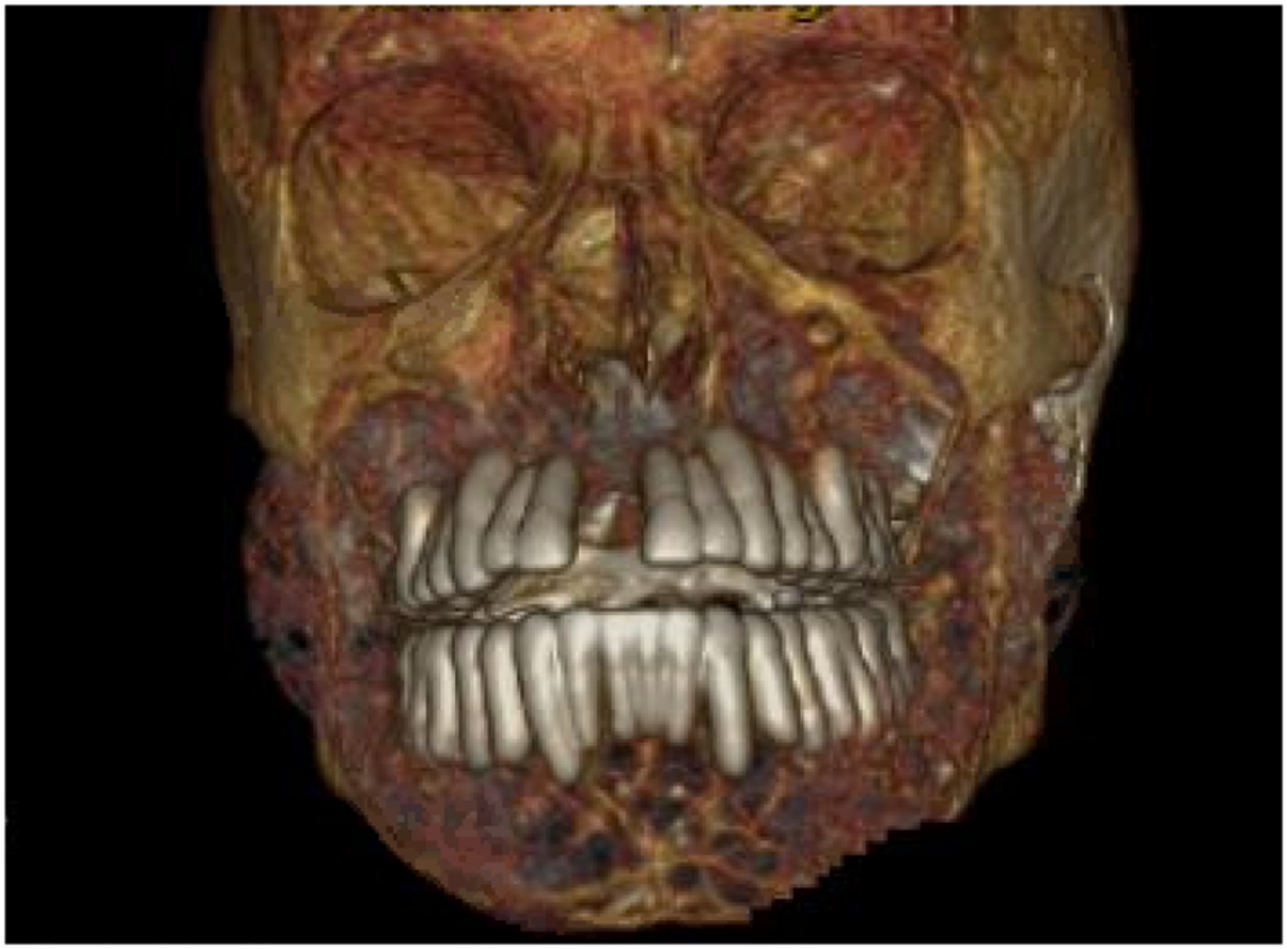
3D rendering of the computed tomography showing abnormal appearance of the maxilla, mandible and the rest of facial bones.
